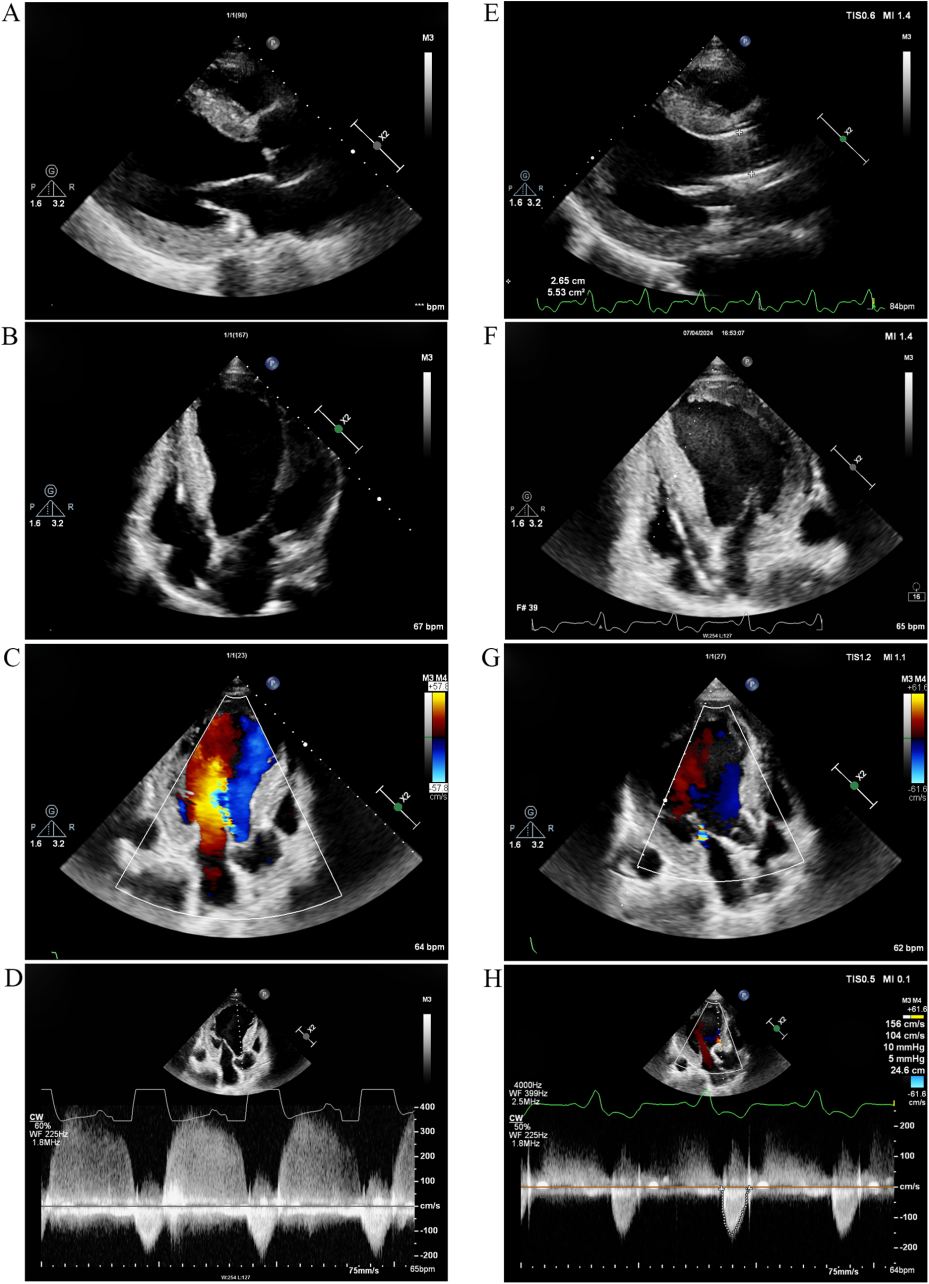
TTE 2 years after ERT and 1 month after TAVR. (A) Parasternal long-axis view of the left ventricle and (B) Apical four-chamber view 2 years after ERT. The left ventricle was significantly enlarged, and the left ventricular ejection fraction decreased to 27.4%. (C) Color Doppler flow imaging and (D) continuous wave Doppler 2 years after ERT revealed echo enhancement of the aortic valve and severe aortic regurgitation. (E) Parasternal long-axis view and (F) apical four-chamber view demonstrated improvement in left ventricular chamber diameter and enhancement of cardiac systolic function 1 month after TAVR. (G) Color Doppler flow imaging and (H) Continuous wave Doppler indicated that aortic regurgitation improved 1 month after TAVR. TTE, transthoracic echocardiography; ERT, enzyme replacement therapy; TAVR, transcatheter aortic valve replacement.
